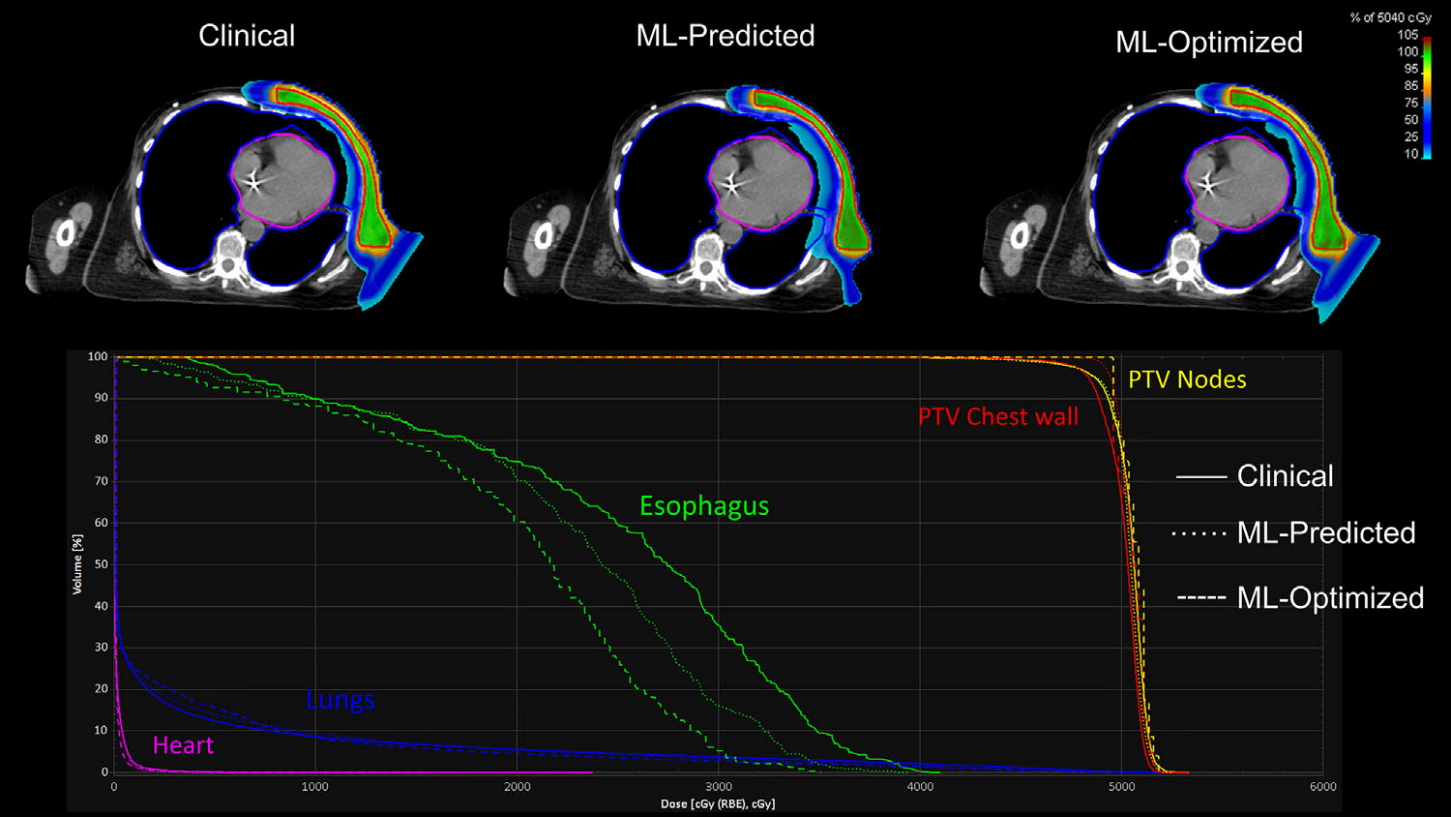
Axial CT slice with distributions of the clinical, ML‐predicted, and ML‐optimized treatment plans for a chest wall target (red contour) (upper) and corresponding target and OAR DVH curves (lower).
